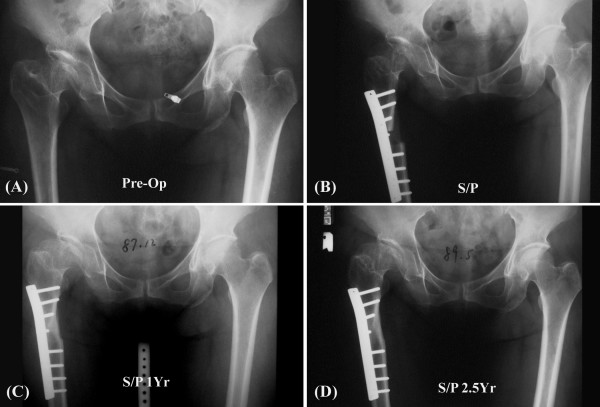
Radiographs of a dysplasia patient who received an L-osteotomy on Jan. 12, 1998. (A) Shortening of the femoral neck and relative overgrowth of the greater trochanter, (B) Radiologic appearance after the L-osteotomy and the postoperative results at (C) 1 year and (D) 2.5 years.
